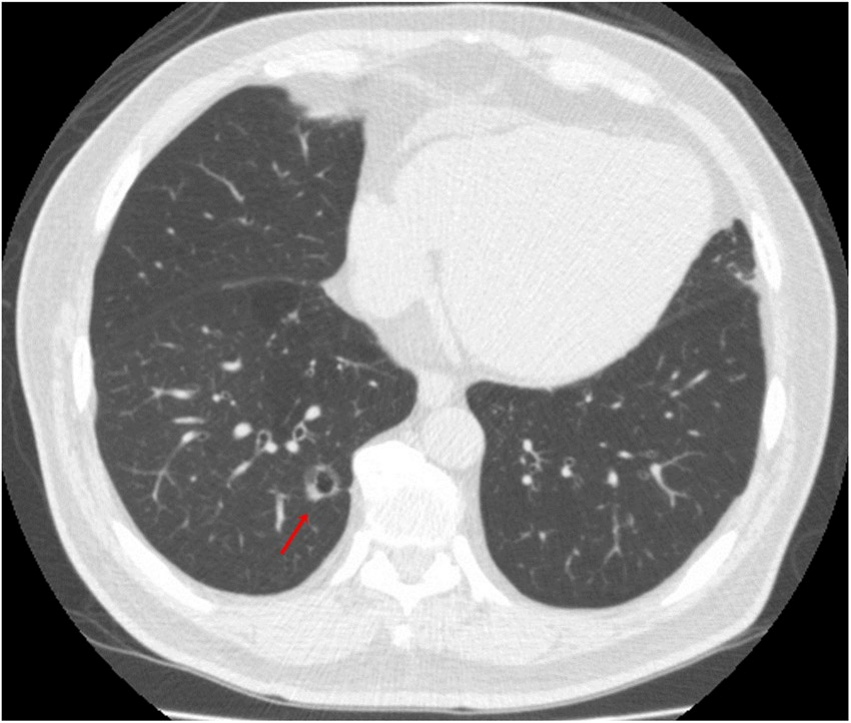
An example of subsolid nodule with abnormal cystic-like airspace according to the SSN subclassification. A 55-year-old man had a 1.1 cm part-solid nodule in RLL. The axial CT image showed an abnormally dilated cystic-like airspace inside the lesion. The patient underwent video-thoracoscopic wedge resection of RLL. Further pathologic report demonstrated invasive pulmonary adenocarcinoma in RLL, Stage 1. Abbreviations: SSN = subsolid nodule; RLL = right lower lobe.
